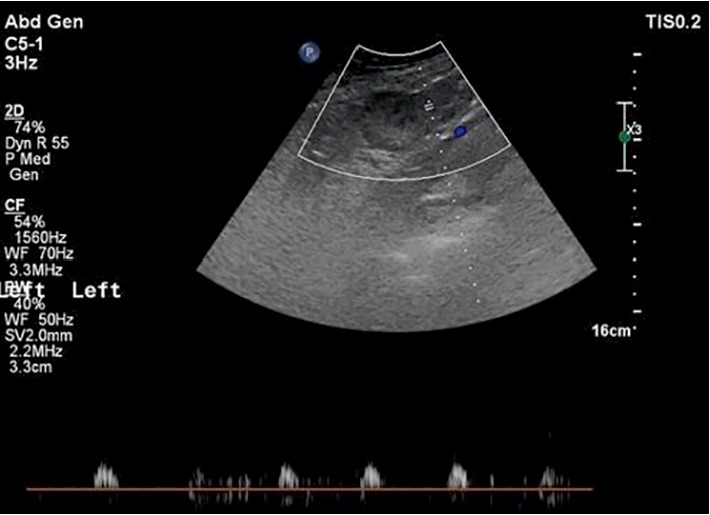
Absent Doppler flow to the enlarged left ovary.
